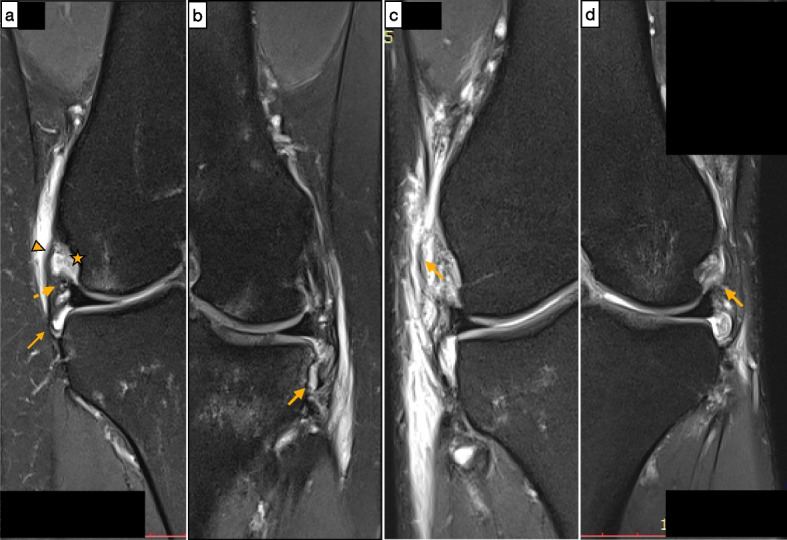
a Coronal T2-weighted images with fat saturation shows the normal femoral portion (arrowhead), meniscal portion (dotted arrow) and tibial portion (arrow) of anterolateral ligament; popliteus tendon (star); b Segond avulsed bone fragment (arrow); c femoral portion of ALL presenting abnormal signal and irregular aspect of its fibers (arrow); d meniscal portion of ALL presenting abnormal signal and irregular aspect of its fibers (arrow)
